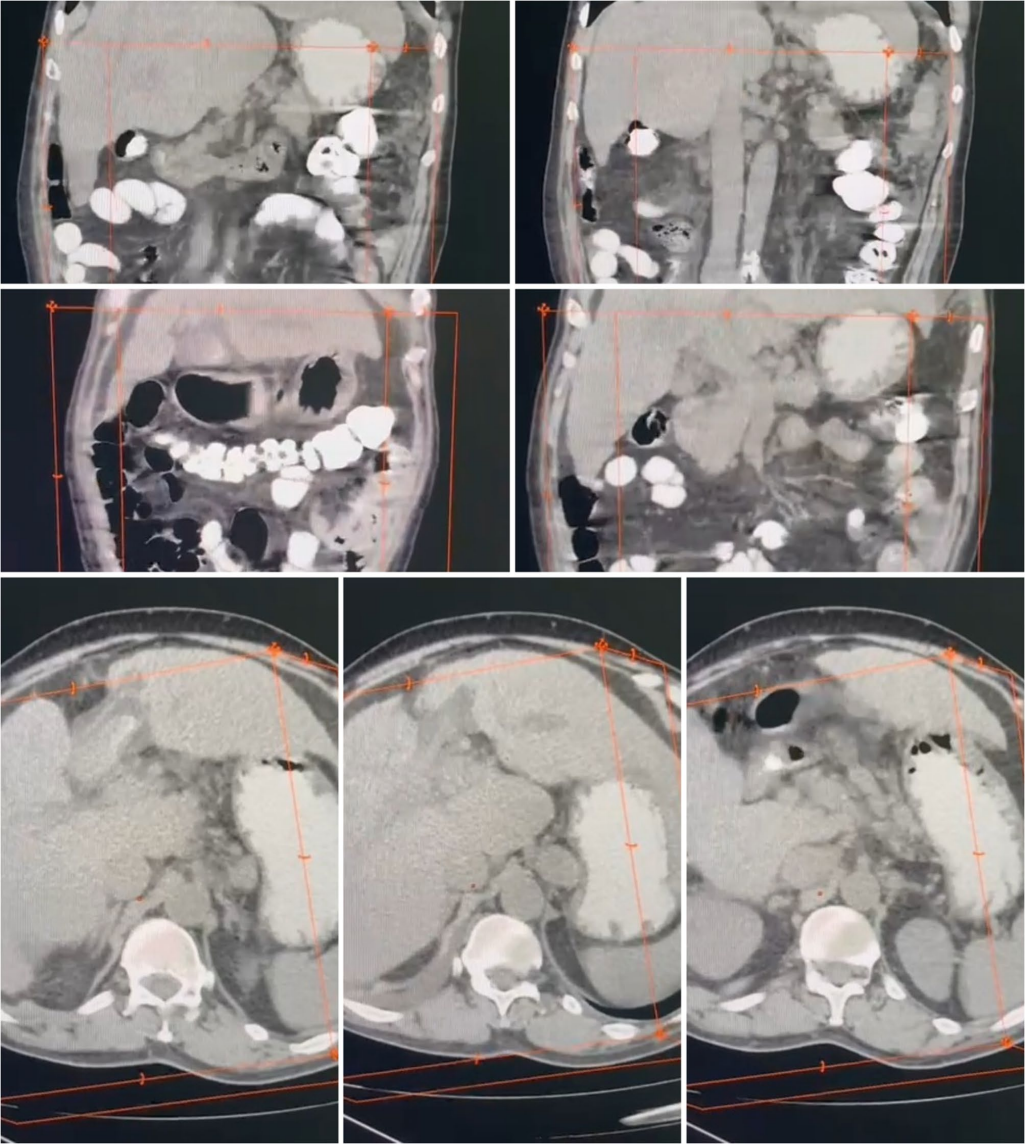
The computed tomography (CT) Cuts delineated an anatomical positioning of the gallbladder, indicative of a true left-sided configuration
